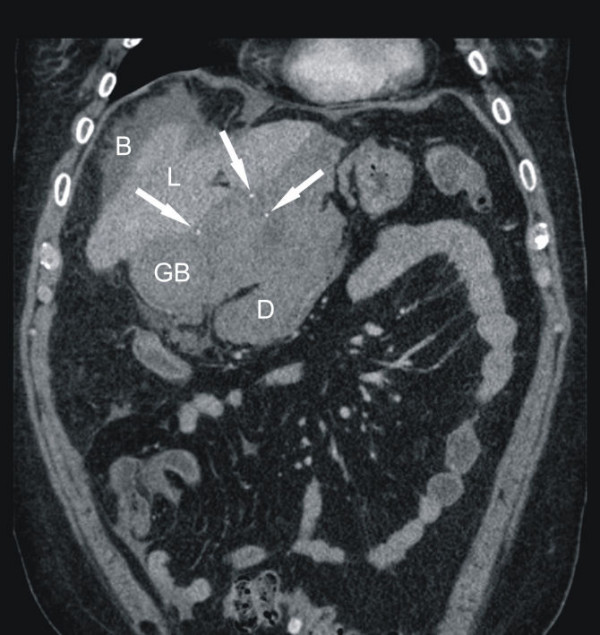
Computed tomography (CT) of the abdomen (coronal reformation). L = liver, GB = gallbladder, D = duodenum, S = spleen, B = blood. Several calcified stones are appreciated outside the gallbladder (solid arrows in figure 2b). Notice also progredient hyperdense fluids surrounding liver and spleen (B), altogether making the diagnosis of free gallbladder perforation obvious.
